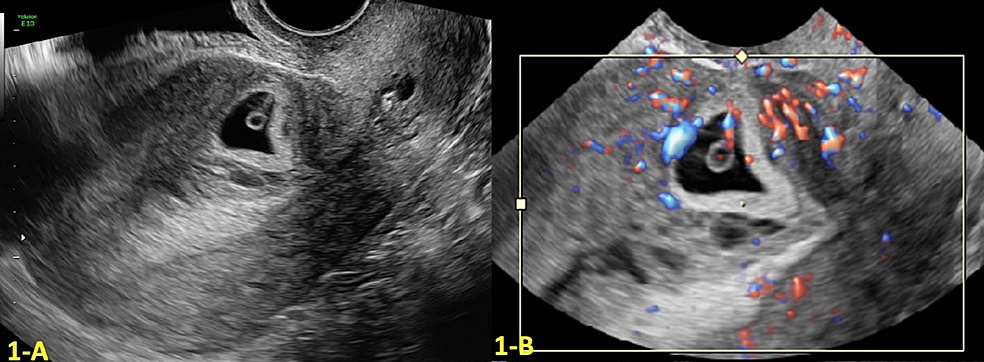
Transvaginal ultrasound (TVUS) findings at six weeks and three days gestational age (GA) A: Transvaginal ultrasound (TVUS) at six weeks and three days gestational age (GA) showing a low-lying gestational sac at the location of the cesarean scar (CS) scar with an empty uterine fundus and cervical canal. B: Color Doppler at six weeks and three days GA showing marked peritrophoblastic flow around the gestational sac consistent with cesarean scar pregnancy (CSP).
